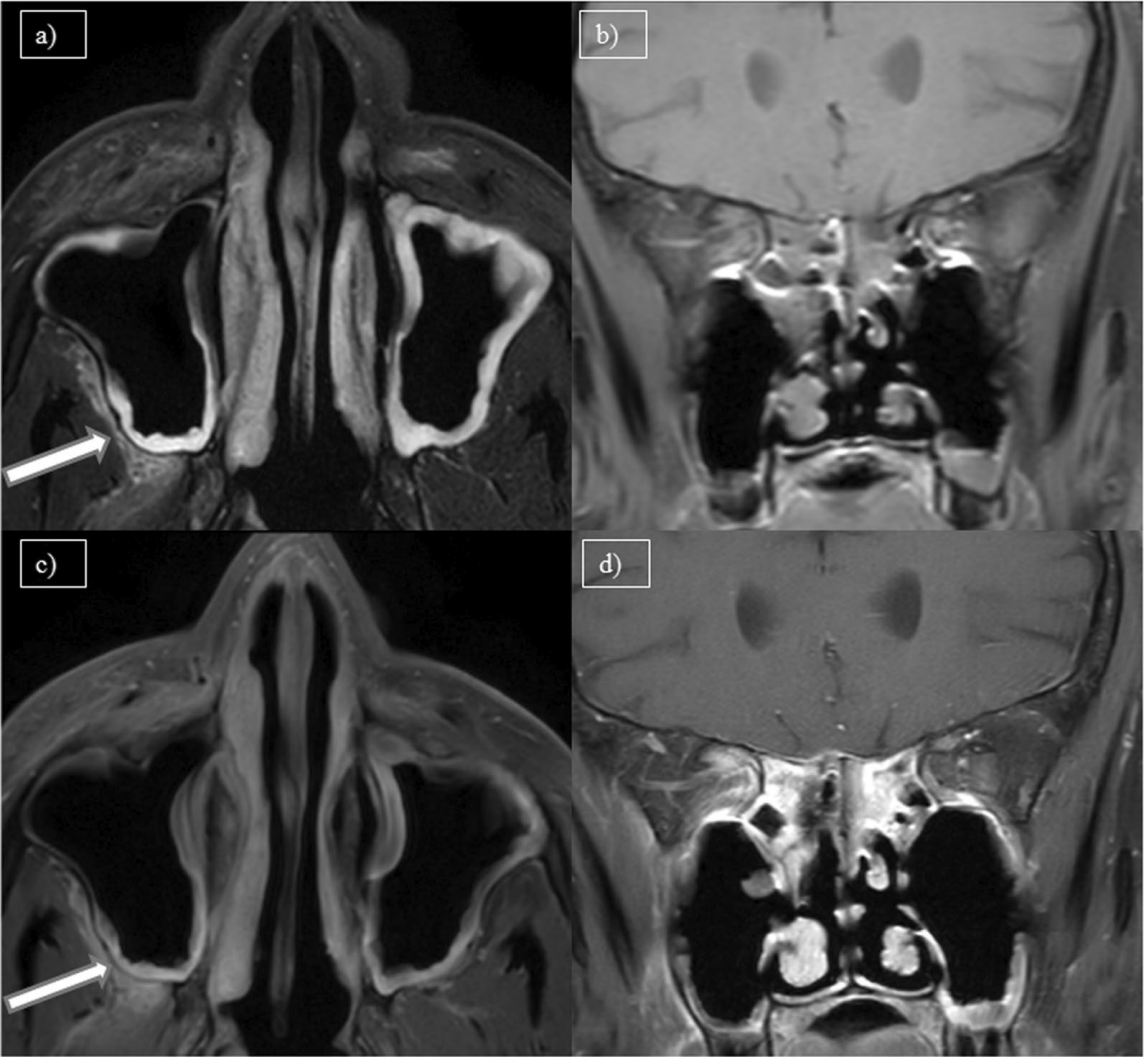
a MRI Axial T2W-FS, b coronal pre-contrast T1W-FS, c and d axial and coronal post-contrast T1W-FS images of a 52-year-old female patient with complaints of headache and rhinorrhea showing bilateral maxillary sinusitis with T2 hyperintense periantral space fat stranding which is showing significant post-contrast enhancement (arrow)
